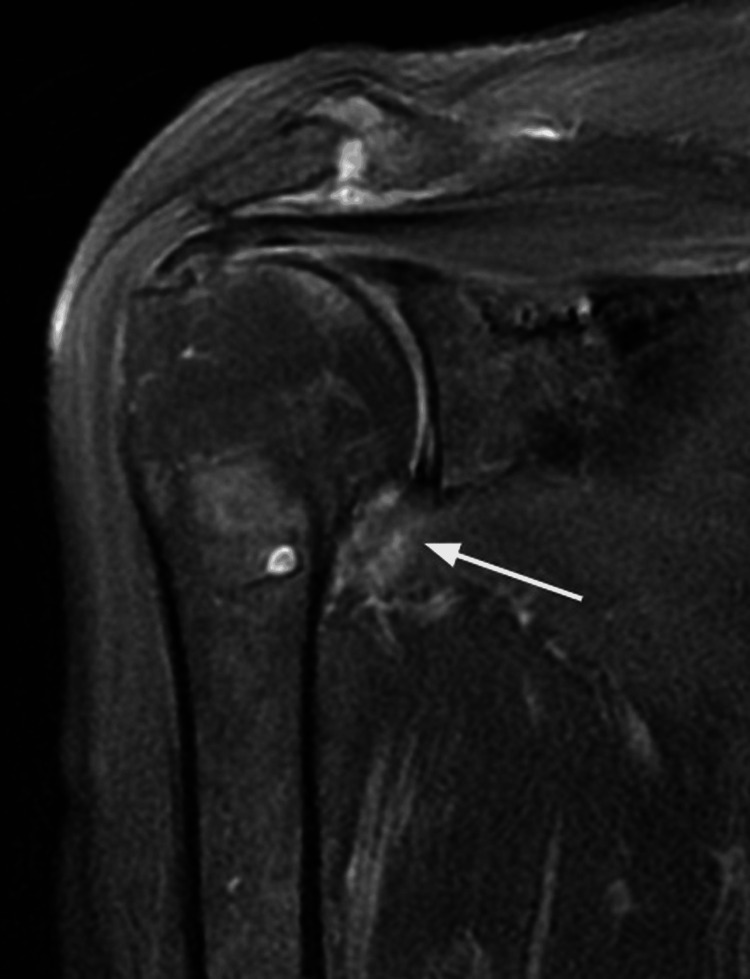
Coronal T2-weighted MRI. The white arrow indicates edema in the axillary recess.
